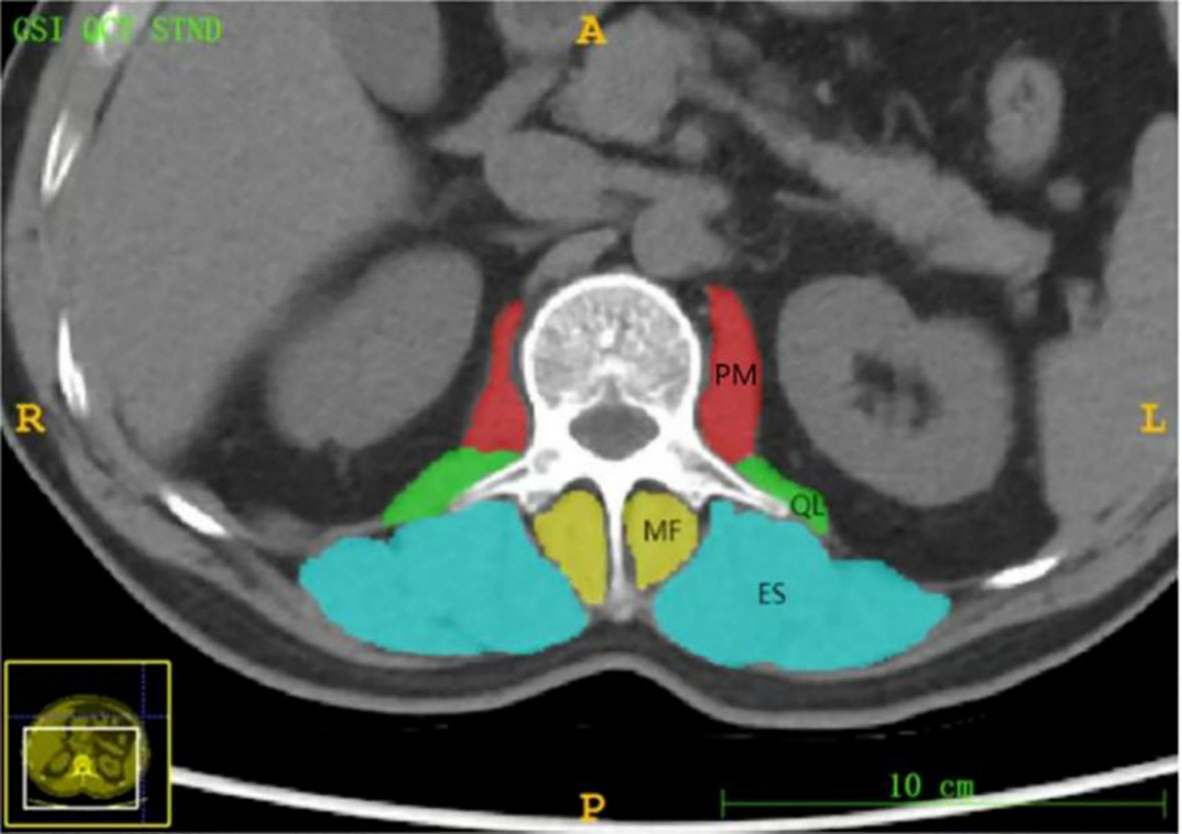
Axial level CT image of the L 1 spinous process. The paravertebral muscles (including the psoas major (PM), quadratus psoas (QL), erector spinalis (ES) and multifidus (MF)) are outlined as shown.
